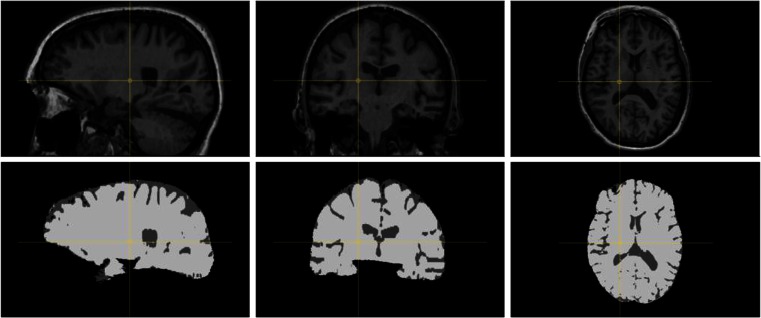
Fig. 3 T1-weighted image (*first row*) and result after segmentation in brain tissue and CSF (*second row*). The brain is depicted in sagittal, coronal and axial orientation
